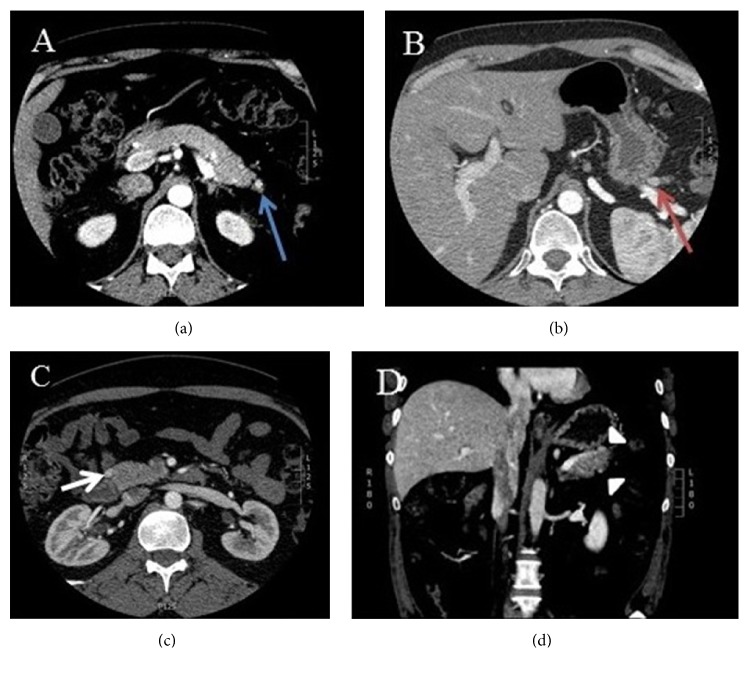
CT of the pancreas showing three hyperenhancing lesions. (a) 4 mm lesion in the inferior aspect of the pancreatic tail (blue arrow). (b) 9 mm lesion in the superior aspect of the pancreatic tail (red arrow). (c) 10 mm lesion in the pancreatic head (white arrow). (d) Coronal view revealing both pancreatic tail lesions (arrow heads).
